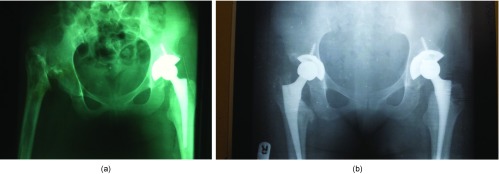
(a) Preoperative X-ray with resection arthroplasty of the right hip and THR prosthesis insitu left hip. (b) Post operative X-ray after THR of the right hip.
